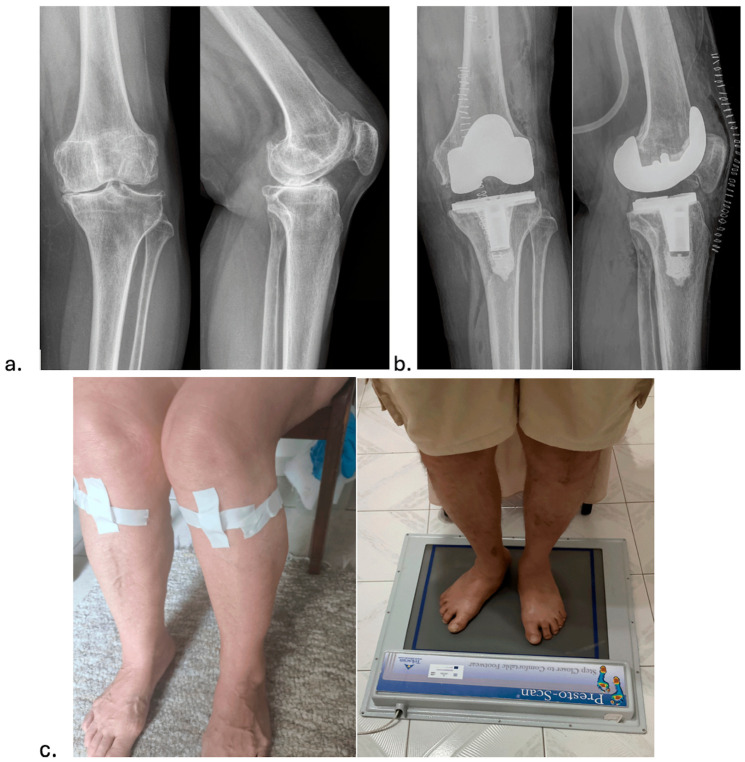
An exemplary patient case showing (a) preoperative and (b) postoperative knee X-rays of the procedure, which was performed using a gap-balancing technique within the framework of functional alignment surgery, as well as (c) pictures from the gait analysis (preoperative sensor placement around the knees and body weight distribution between legs measured with the patient standing barefoot on a posturographic platform.
